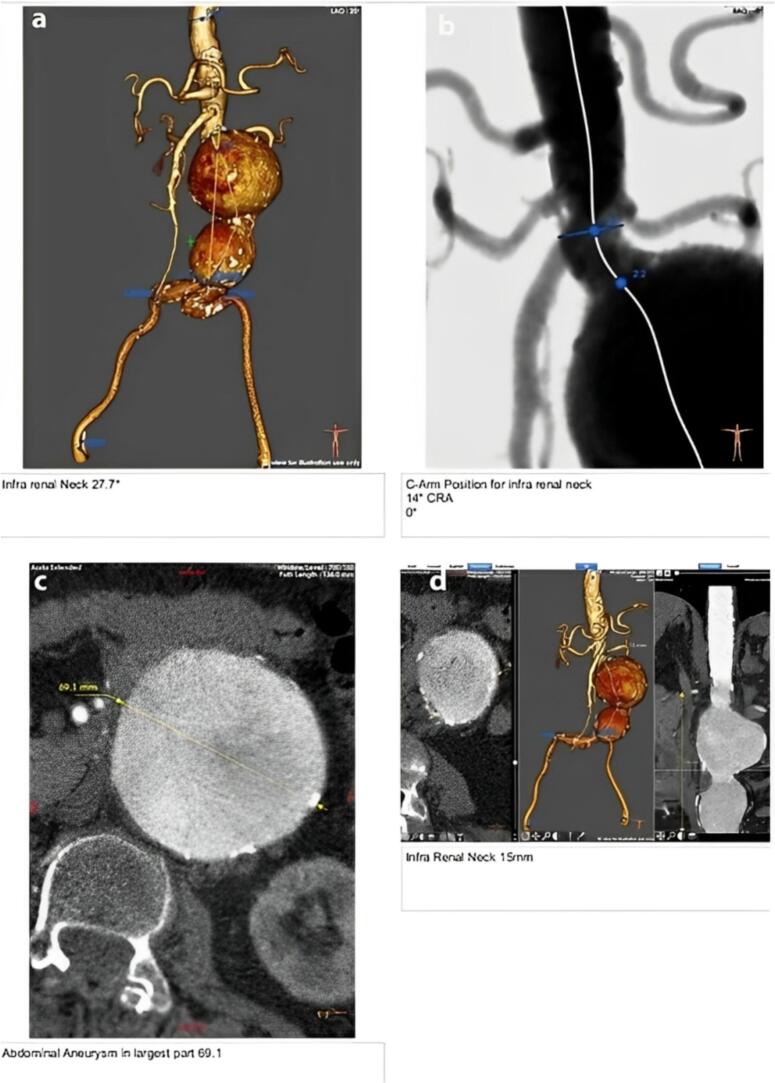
Various Viewpoints of the Aneurysm, including (a) a 3D CT angiographic reconstruction showing an infrarenal abdominal aortic aneurysm with a neck length of 27.7 mm; (b) a fluoroscopic image with optimal C-arm positioning (14° cranial angulation, 0° rotation) for assessment oh = f the infrarenal neck during endovascular planning; (c) an axial CT image demonstrating the maximal transverse diameter of the aneurysmal sac; and (d) #d post-processing and coronal CT images showing an infrarenal neck length of 15 mm, providing crucial information for EVAR feasibility and endograft selection.
